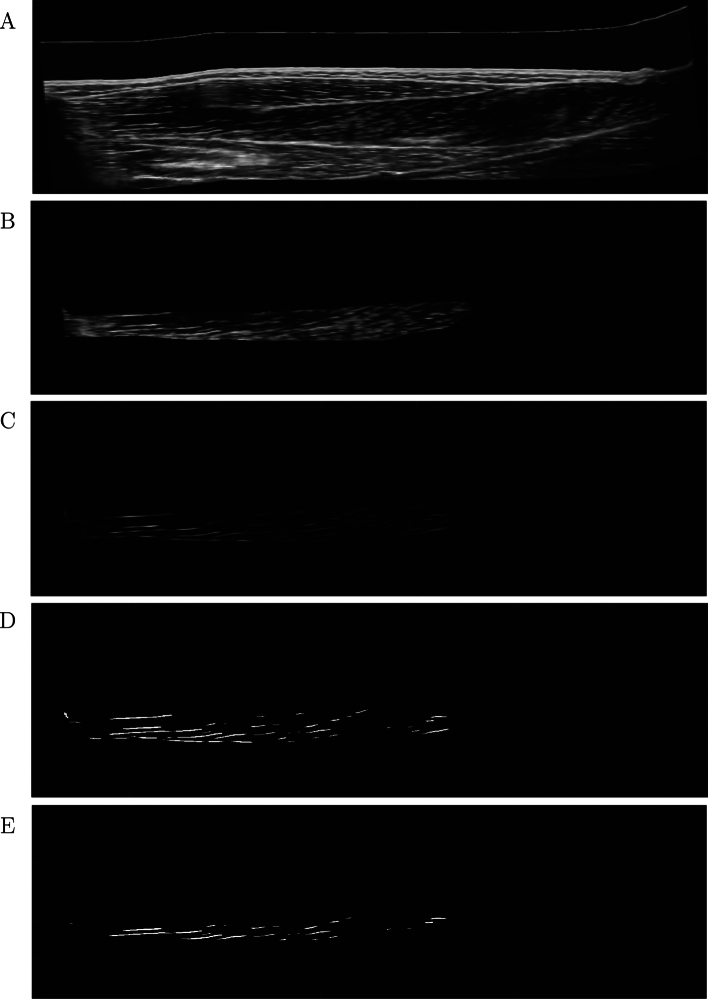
Workflow for fascicle detection from 3D ultrasound images. A Sagittal image slice of reconstructed volume rotated into muscle coordinate system, B masking of muscle compartment, C after applying MVEF, D binarizing filtered image and E shrunk masked
